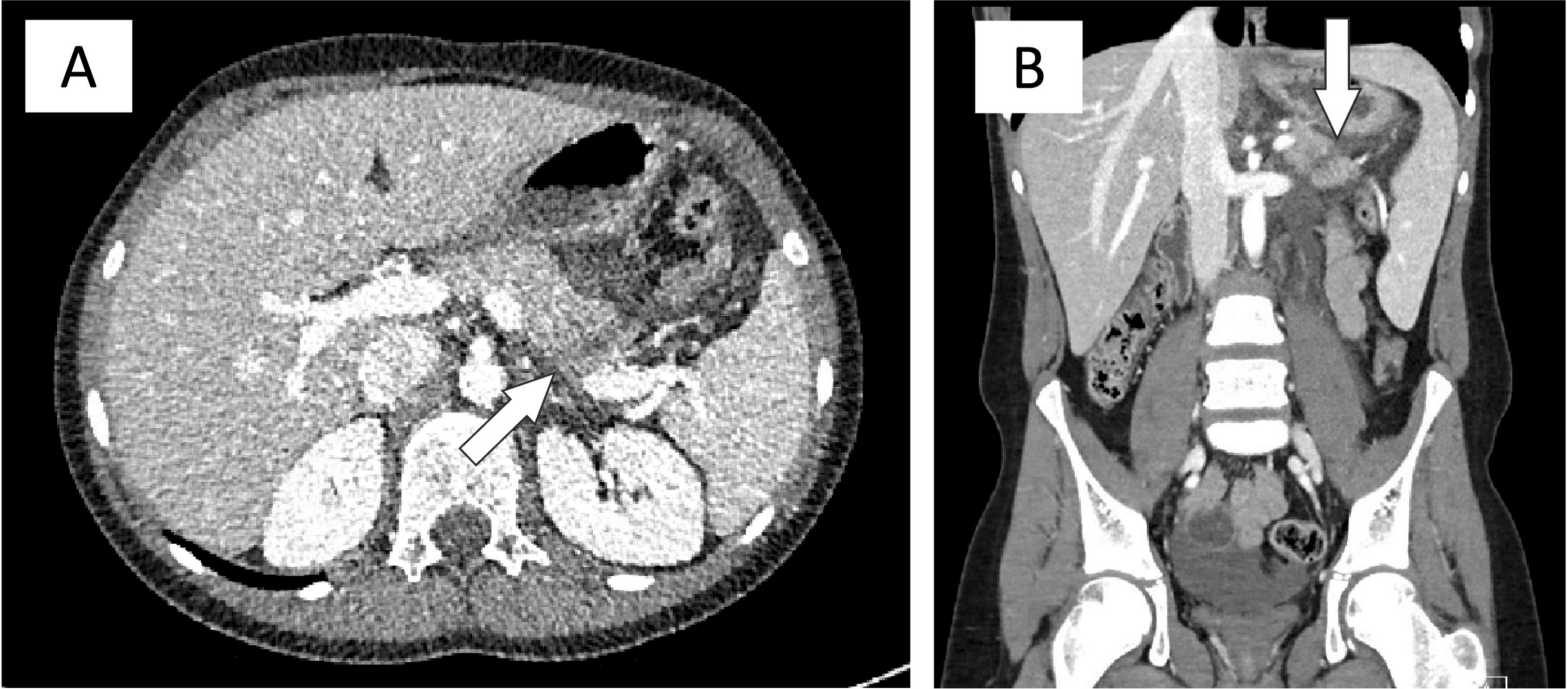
Case 3: Abdominal CT with contrast. (A) Axial view. A laceration near the junction of the pancreatic body and tail involving the majority of the pancreas (white arrow), with possible pancreatic duct involvement, consistent with a grade III pancreatic injury. Peripancreatic and retroperitoneal fluid was also visualized, extending inferiorly toward the pelvis. (B) Coronal view.
